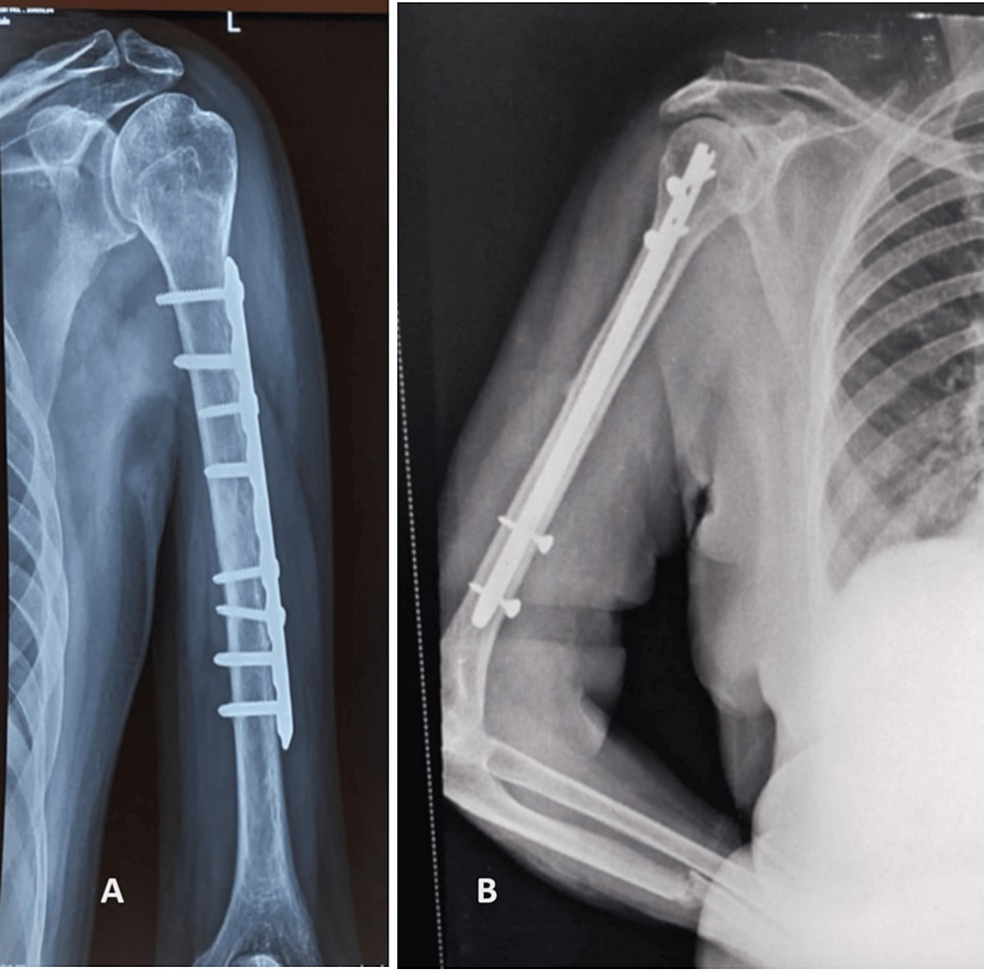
Six-month postoperative radiographs (A) Six-month follow-up radiograph showing a union at the fracture site after performing dynamic compression plating; (B) six-month follow-up radiograph showing union at the fracture site after performing interlock nailing
